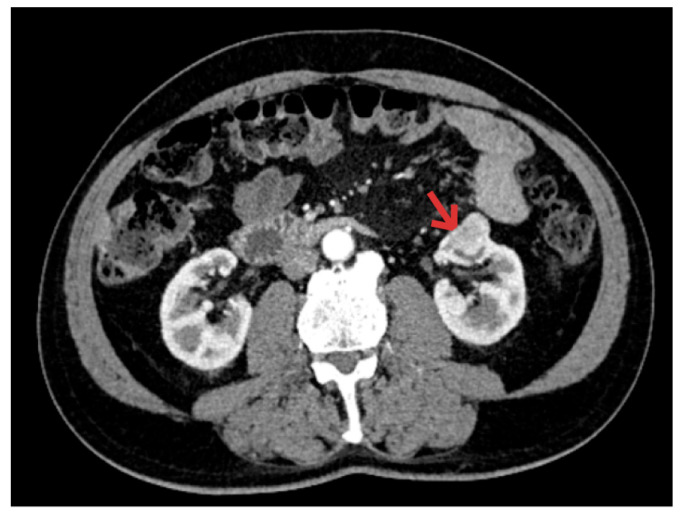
CT image—arrow indicates the renal tumor.
